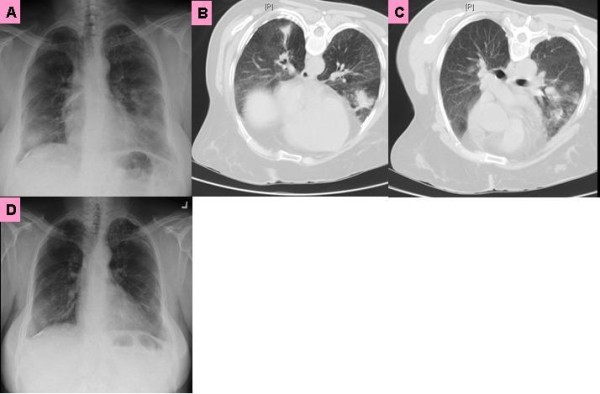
A. Chest X-ray shows fibrocalcific changes, right lower lobe mass. B and C: Chest computed tomography (CT) scan shows pleural scarring in multiple foci, throughout both lung fields with early mediastinal invasion. Right lower lobe mass (biopsy site). Chest X-ray at four month shows diminished right lower lobe opacity.
